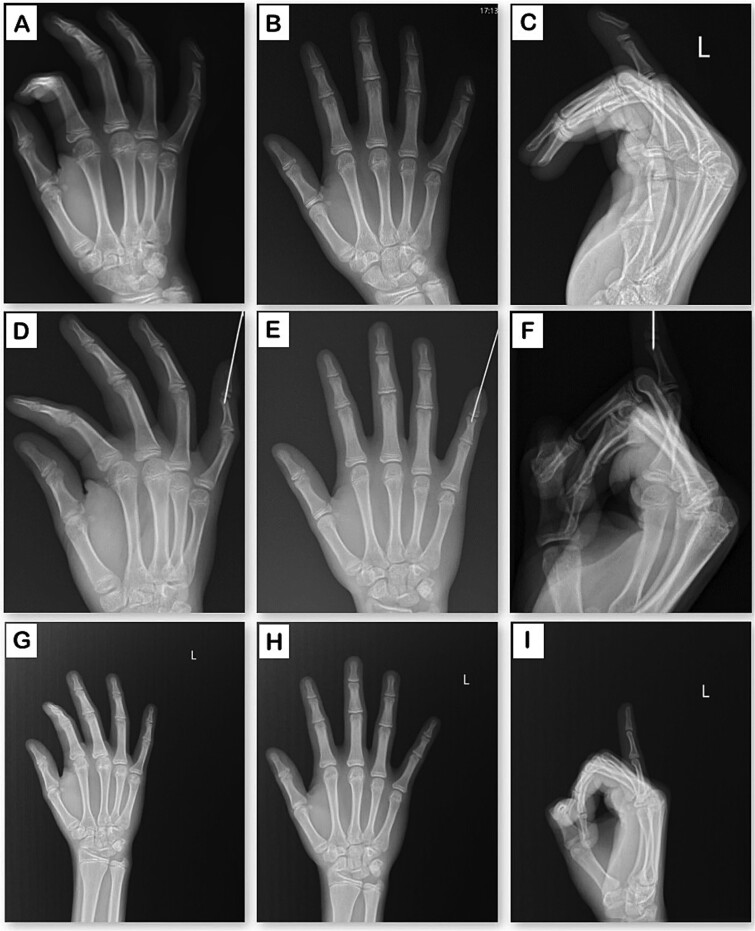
Radiographic evaluation of the hand in three planes. (A–C) Preoperative X-rays: oblique, anteroposterior, and lateral views. (D–F) Postoperative X-rays following surgical intervention: oblique, anteroposterior, and lateral views. (G–I) X-rays at 10-month follow-up: oblique, anteroposterior, and lateral views.
